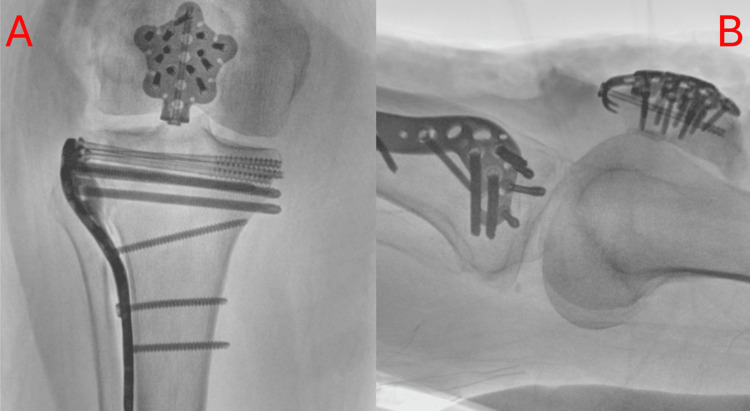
Intraoperative anterior to posterior (a) and lateral (b) X-rays of patellar fixation A) Plain intraoperative anterior to posterior radiograph of the patient's right knee. This image shows the patellar fracture fixed with a plate and screws. It also shows the tibial plateau fracture fixation, seen in Figure 2; B) Plain lateral intraoperative radiograph of the patient's right knee. This image shows the patellar fracture fixed with plate and screws. It also shows the tibial plateau fracture fixation, seen in Figure 2.
